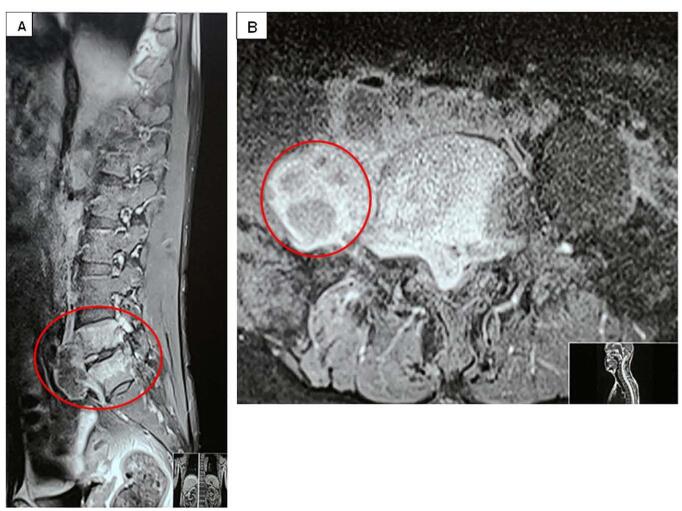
T2 weighted MRI of spine: (A) sagittal cut and (B) axial cut showing signal changes in the L5 vertebral body and multifocal abscesses (rings).
